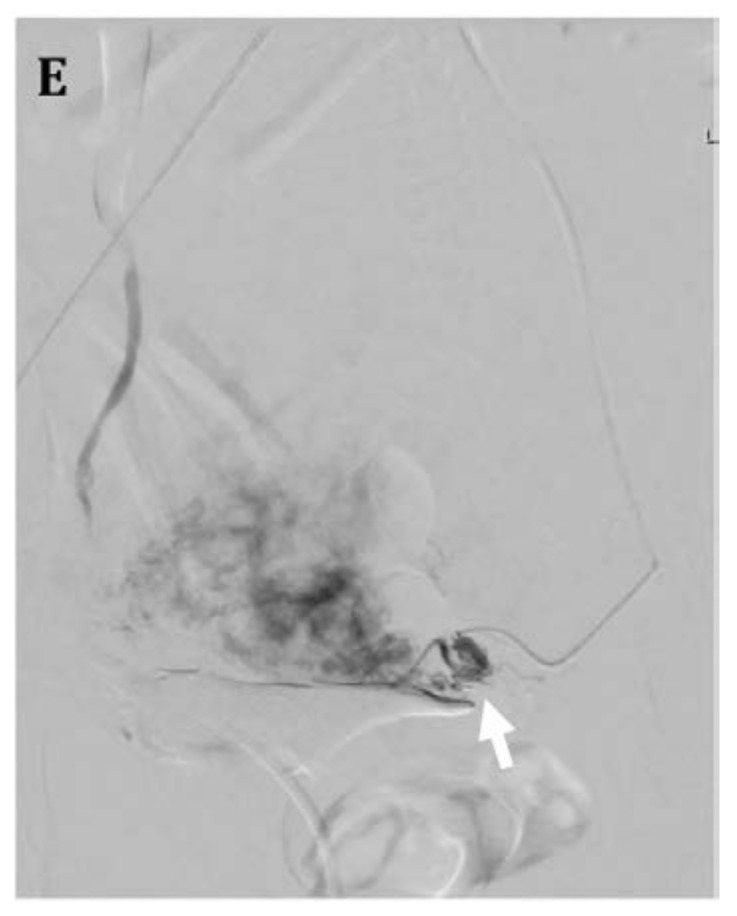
Thirty-five-year-old female with placenta percreta. (A) Ultrasound at 19 weeks of gestation shows an abnormal placenta with multiple placental sonolucencies and focal bulging of placental tissue into the bladder (arrow). (B) Sagittal FIESTA MR image shows no discernable fat plane between placental tissue and bladder serosa (arrowhead), suspicious for bladder wall invasion. (C) Pre-hysterectomy pelvic angiogram shows a large hypervascular placenta (arrows). (D) Selective catheterization of the right uterine artery shows an enlarged right uterine artery (arrow) with marked placental hypervascularity. Embolization of bilateral uterine arteries was performed with 500–700-micron polyvinyl alcohol particles until stasis. (E) Due to concern for placental invasion of the bladder, the left vesicular artery was also selectively catheterized (arrow) and embolized using 500-micron polyvinyl alcohol particles. Bilateral internal iliac artery occlusion balloons were left in place. Patient ultimately underwent hysterotomy with delivery of a non-viable fetus at 22 weeks; hysterectomy was aborted due to significant pelvic side wall involvement. Patient has been subsequently followed with serial MR imaging for retained placenta.
